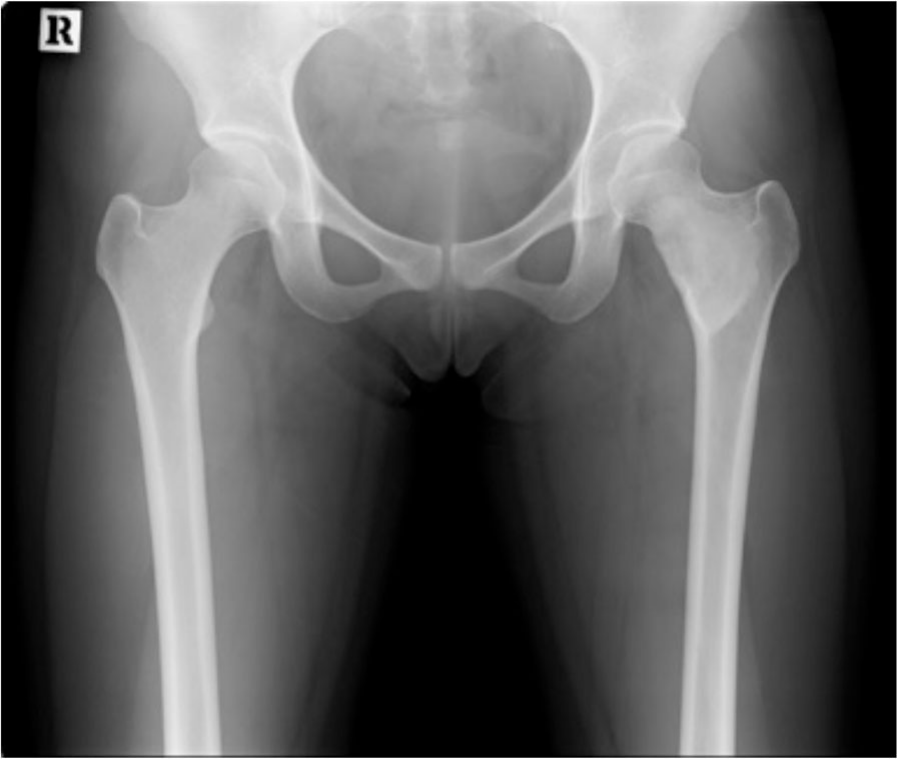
X-ray of the hip, demonstrating ground glass lesion in left neck of femur
